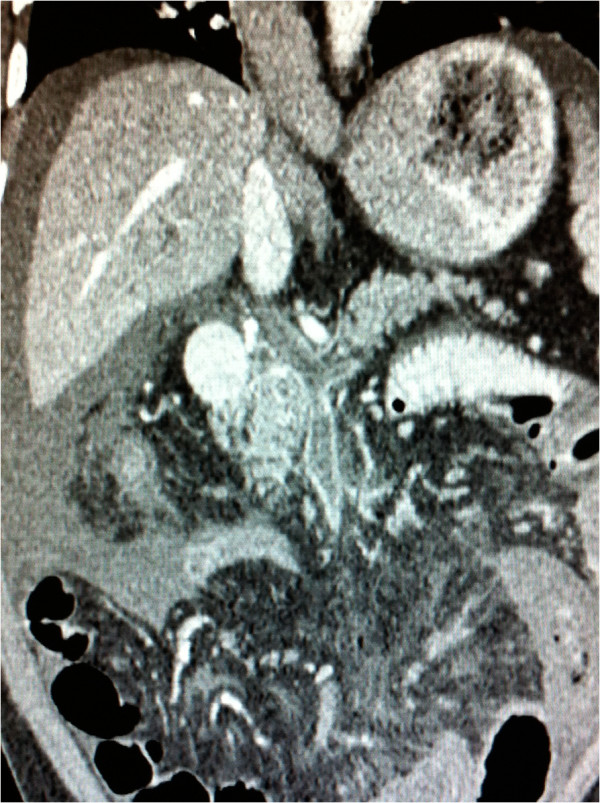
Longitudinal computed tomography scan of the patient’s abdomen with contrast enhancement taken in the axis of the portal vein showing extensive thrombosis involving the superior mesenteric, splenic and portal veins.
